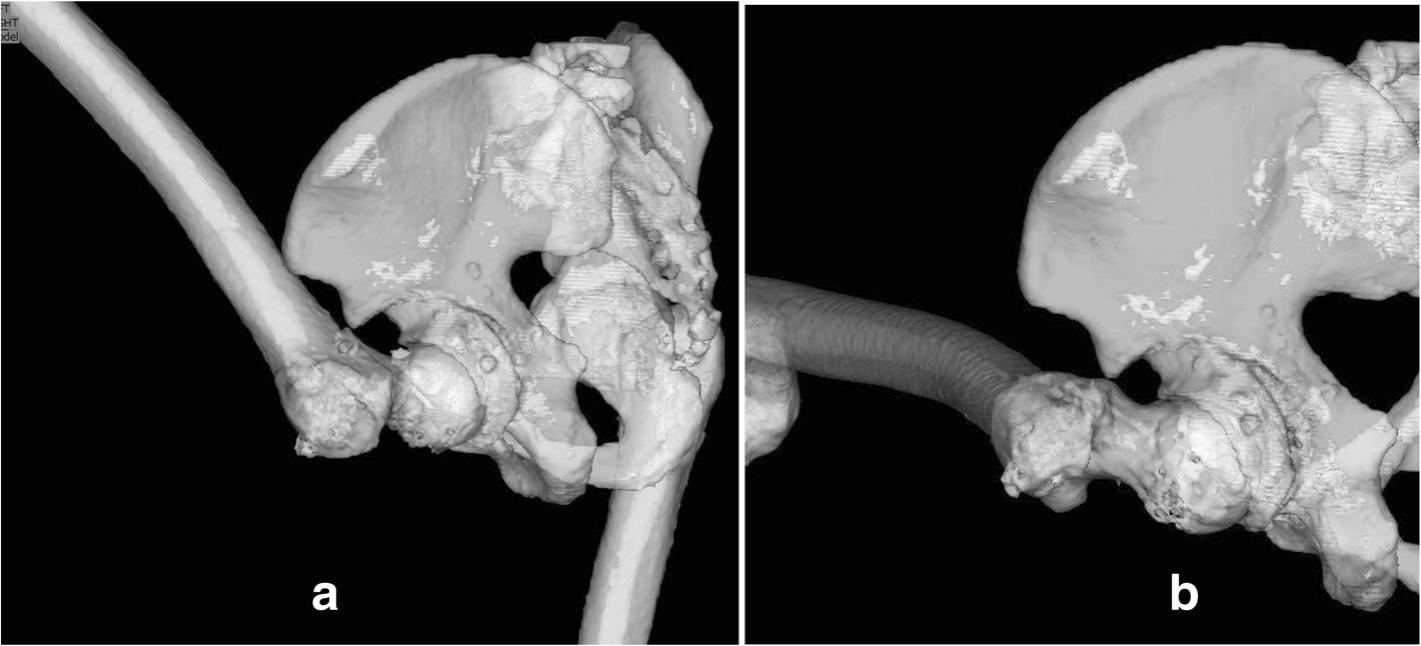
a, b Based on the postoperative CT images and using the ZedHIP® software, we assessed that this patient would be capable of at least 126° of hip flexion and at least 20° of adduction and 20° of internal rotation at 90° of flexion until impingement occurred between the anterior osteotomized fragment and the anterior portion of the femoral neck
